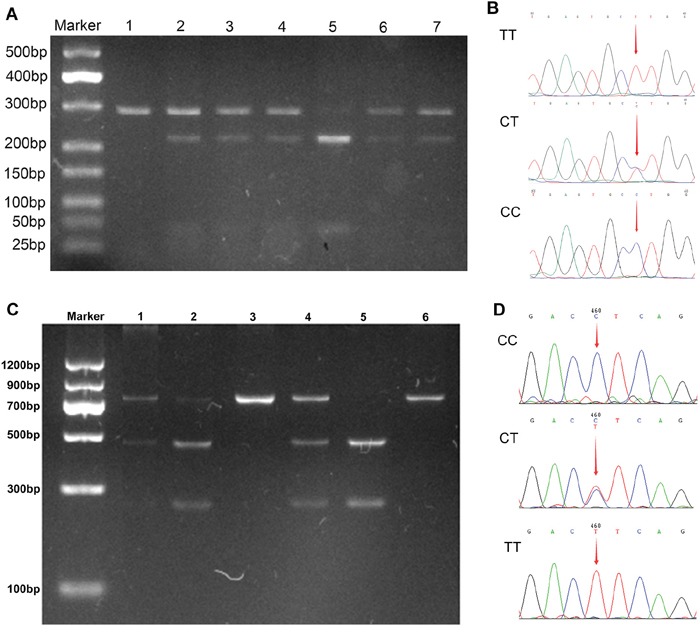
PCR-RFLP and sequencing assay for analyzing the -1535C>T and -3187G>A polymorphisms A. PCR-RFLP assay for -1535C>T, lane 1: TT(283bp); lane 2, 3, 4, 6, 7: CT (283bp, 218 bp, 65 bp); lane 5: CC (218bp, 65 bp). B. Sequencing assay for -1535C>T, the arrays in panels shown TT, CT, and CC, respectively. C. PCR-RFLP assay for -3187G>A, lane 1, 2, 4: CT (731bp, 468bp, 263bp); lane 3, 6: CC(731bp); lane 5: TT (468bp, 263bp). D. Sequencing assay for -1535C>T, the arrays in panels shown CC, CT, and TT, respectively.
